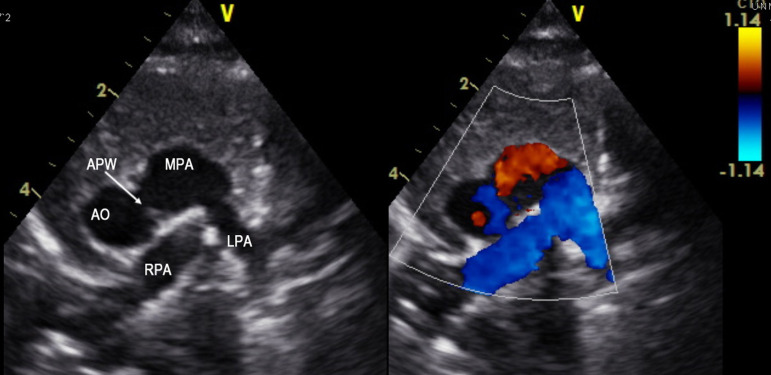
2D echocardiography images showing distal variety of aortopulmonary window with predominant left to right shunt.
